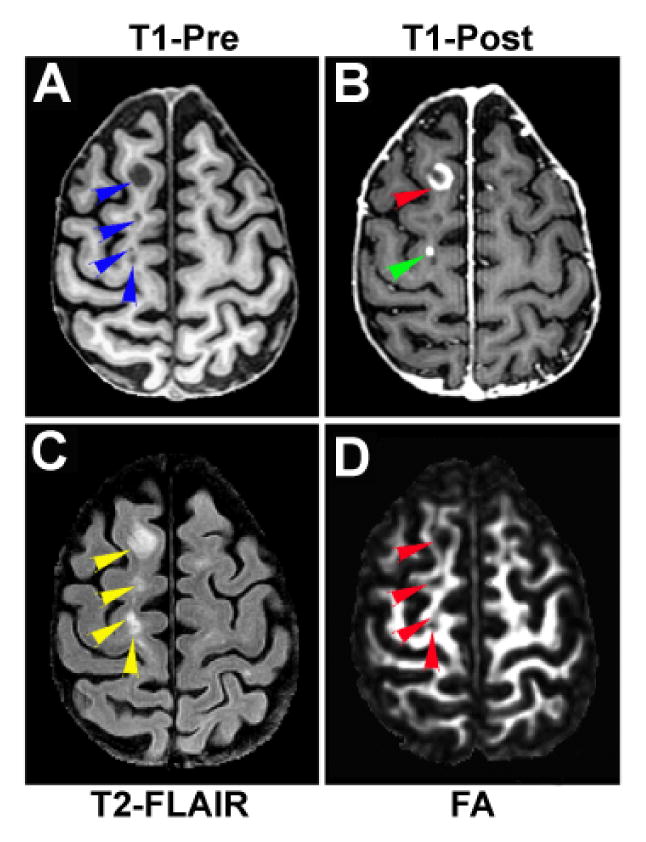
Relationship between T1-enhanced and T1-hypointense region. Some areas withT1-hypointense signal on T1-Pre image (arrowheads in A) concurrently, may be the T1-enhanced regions on T1-Post image (arrowheads in B). Both T1-enhanced and T1-hypointense regions present as hyperintensities on T2-FLAIR image (comparing A, B with C). The reduced FA regions (arrowheads in D) correspond not only to the T1-hypointense lesions, but also to the adjacent active (T1-enhanced) lesions (comparing A, B with D).
